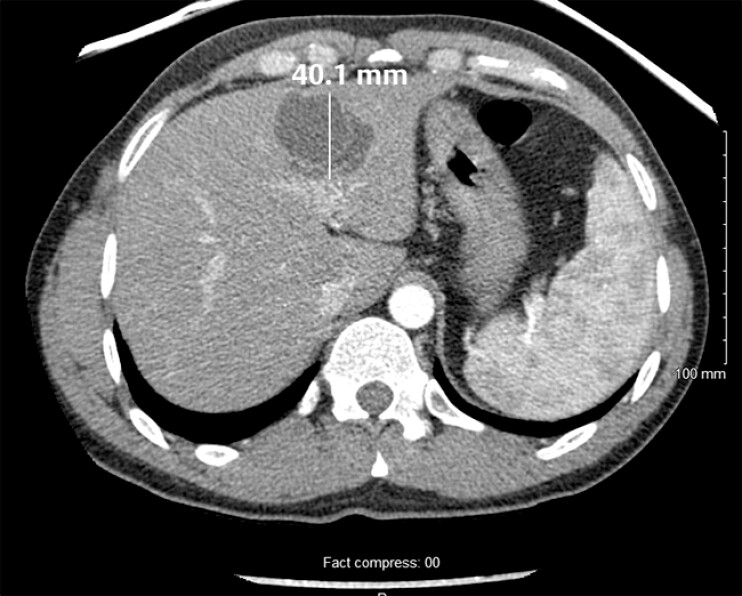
A CT scan showed a multi-lobulated, septated, calcified lesion in the inferior left liver, suggestive of a hepatic cyst. CT, computed tomography.
